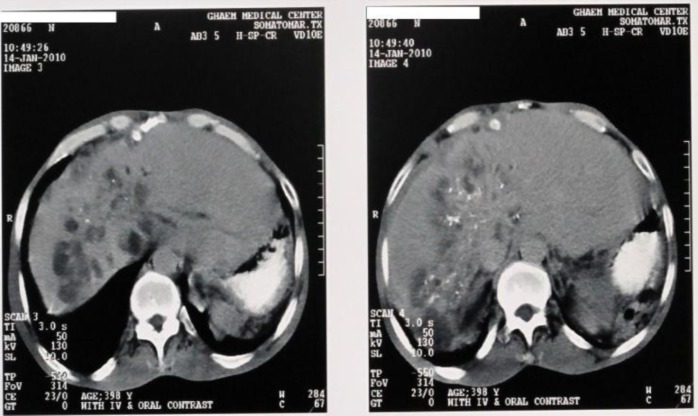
CT scan of the patient suffered from biliopulmonary fistula shows multiple cysts and microcalcifications and plaquelike calcific foci within and around the lesion
